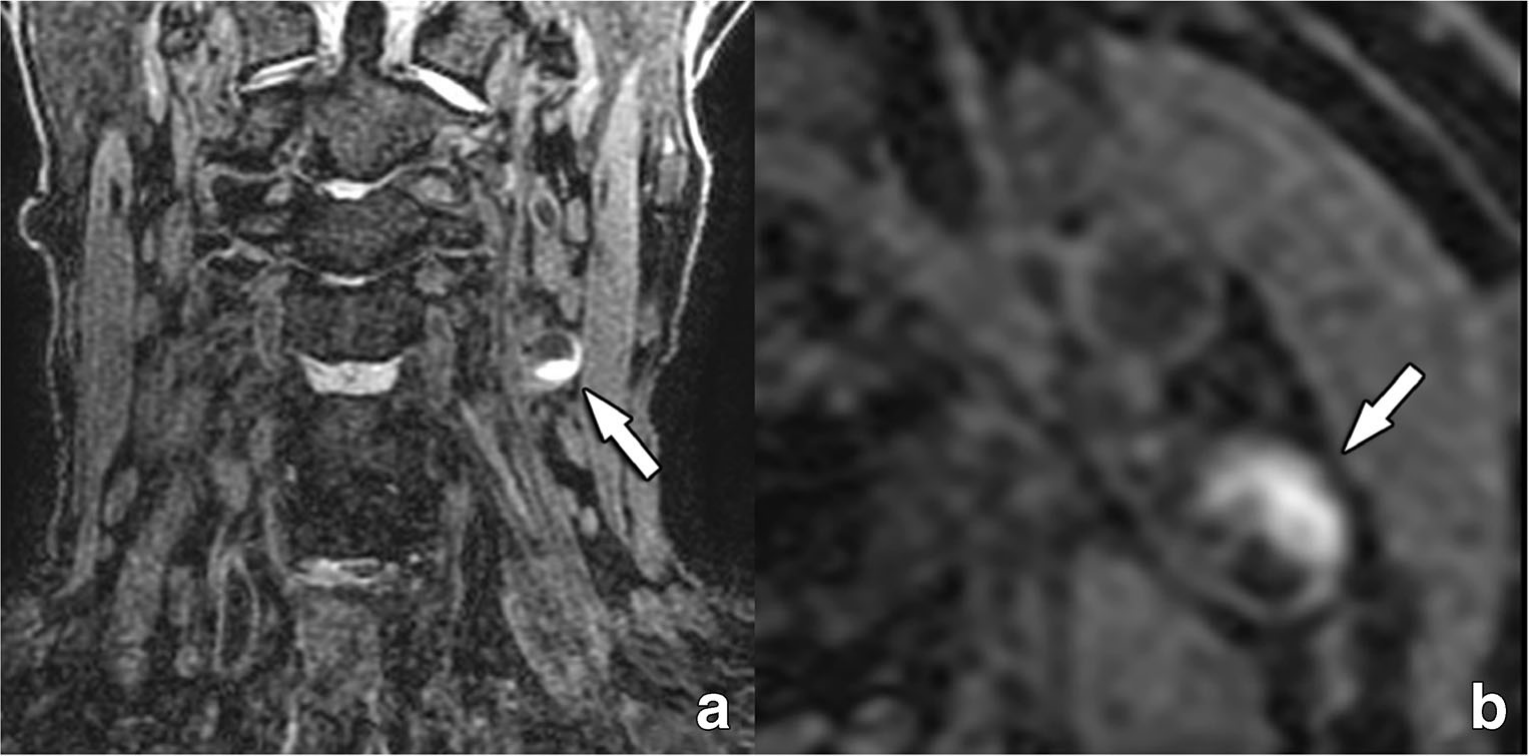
A 83-year-old female with a left-sided stroke and intraplaque hamorrhage as detected with MR. A 3D MPRAGE MR image reveals a high-intensity semi-circumferential plaque in the left internal carotid artery (arrow in a, b), corresponding to intraplaque hemorrhage. The excellent tissue contrast resolution of MR makes it the non-invasive imaging method of choice for the detection of blood degradation products in hemorrhagic plaques. MPRAGE: magnetization-prepared 180° radio-frequency pulses and rapid gradient-echo
